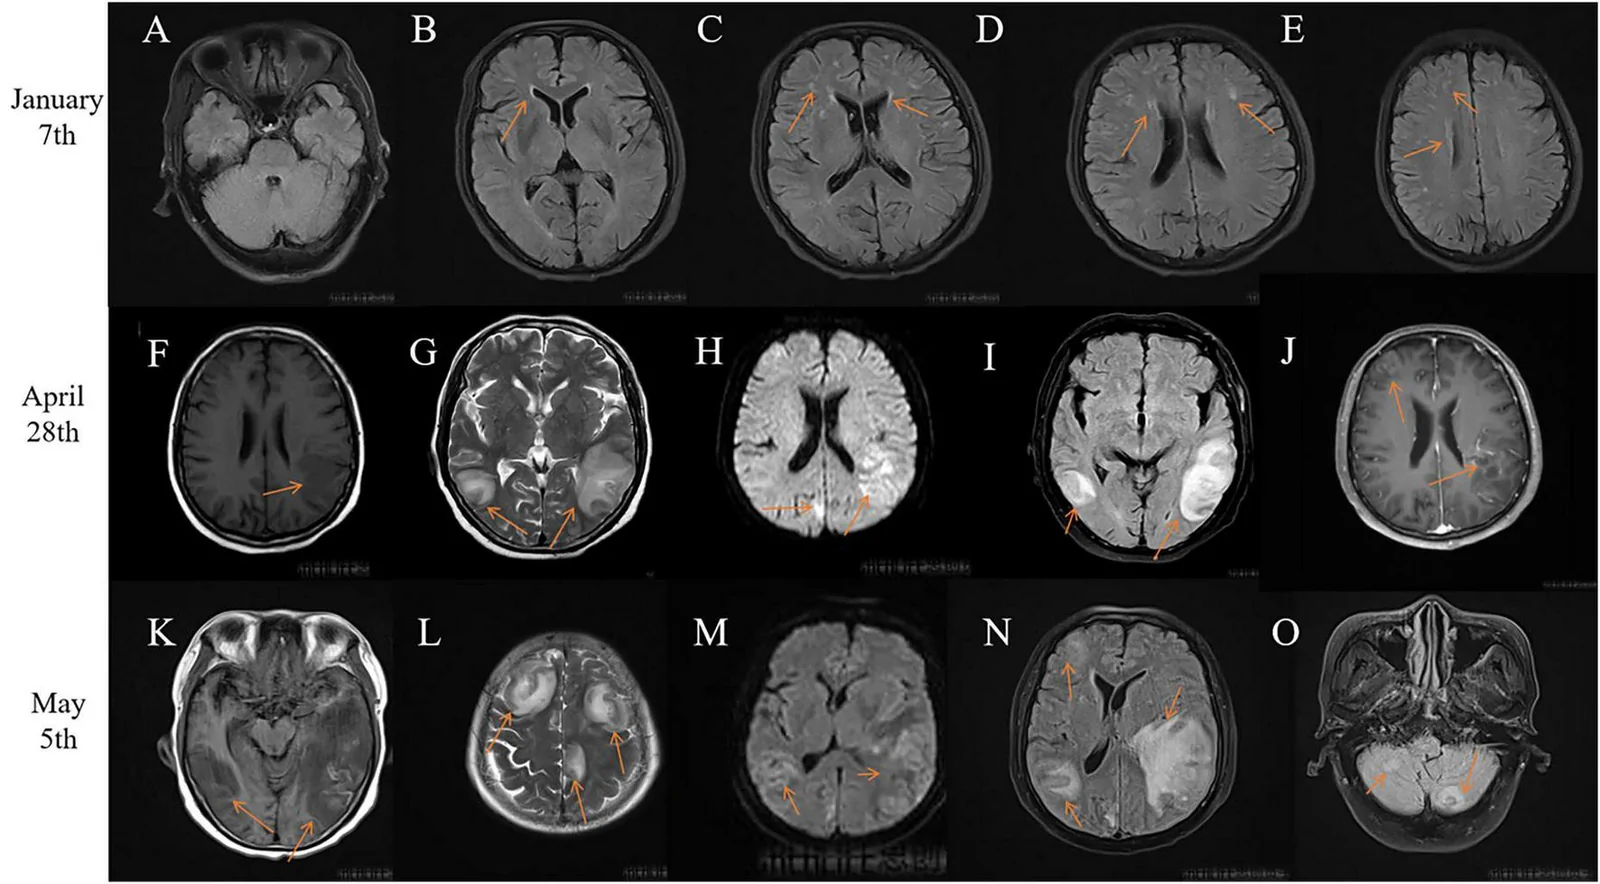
(A–E) Show MRI images from January 7, 2025. (A–E) All five FLAIR images demonstrate ischemic white matter lesions involving the bilateral frontoparietal subcortical white matter, centrum semiovale, and periventricular white matter adjacent to the lateral ventricles, April 28, 2025. (F) The T1-weighted image demonstrates subcortical swelling and abnormal signal intensity in the left cerebral hemisphere. (G) The T2-weighted images demonstrate subcortical swelling and abnormal signal intensity in both cerebral hemispheres. (H) Diffusion-weighted imaging demonstrates multiple patchy and mildly elongated areas of increased signal intensity involving the cortical and subcortical regions of both cerebral hemispheres, accompanied by cerebral parenchymal edema. The largest lesion is located in the left temporo-occipital region and causes compression of the temporal horn of the left lateral ventricle. (I) T2-weighted FLAIR images reveal multiple patchy, mildly elongated hyperintense lesions in the cortical and subcortical regions of both cerebral hemispheres. (J) Contrast-enhanced MRI demonstrated multiple patchy and small ring-enhancing lesions with heterogeneous enhancement in the cortical and subcortical regions of both cerebral hemispheres and in both cerebellar hemispheres, accompanied by associated parenchymal swelling, May 5, 2025 (K–O). (K) Follow-up T1-weighted imaging demonstrates swelling of both cerebral hemispheres, with an increase in the extent of the abnormal signal-intensity lesions compared with the previous examination. (L) T2-weighted imaging revealed multiple areas of abnormal signal intensity in the cortices of both cerebral hemispheres, with an increase in the extent of the lesions. (M) DWI revealed multiple patchy and mildly elongated areas of abnormal signal intensity in the cortical and subcortical regions of both cerebral hemispheres. These lesions were mildly hyperintense on DWI and were accompanied by cerebral parenchymal swelling and increased compression of the temporal horn of the left lateral ventricle. (N) T2-FLAIR imaging revealed multiple patchy and mildly elongated areas of abnormal signal intensity in the subcortical regions of both cerebral hemispheres. These lesions were hyperintense on T2-FLAIR images and had increased in size. (O) T2-FLAIR imaging revealed new lesions in the subcortical regions of both cerebellar hemispheres.
